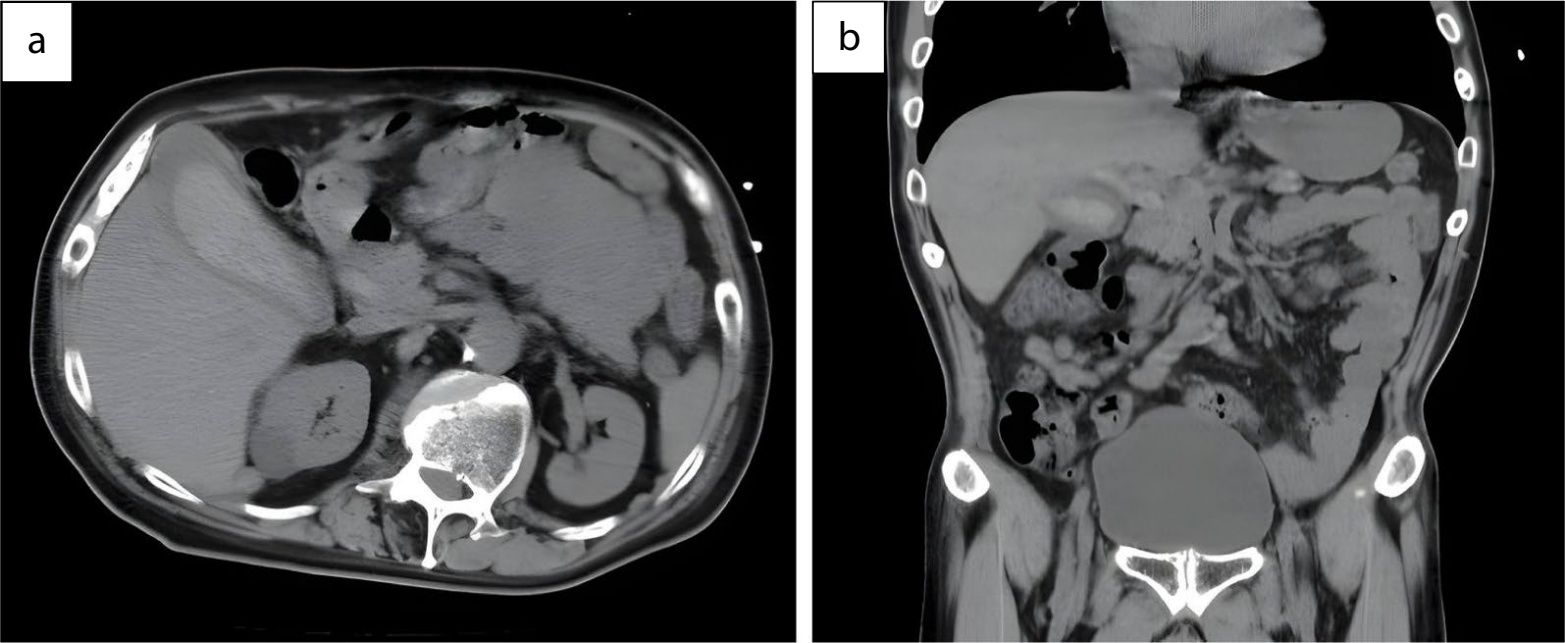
Abdominal CT images. Axial view (a) and coronal view (b) reveal diffuse thickening of the gallbladder wall and a high-density image in the gallbladder and common bile duct, suggesting gallbladder hemorrhage. CT, computed tomography
